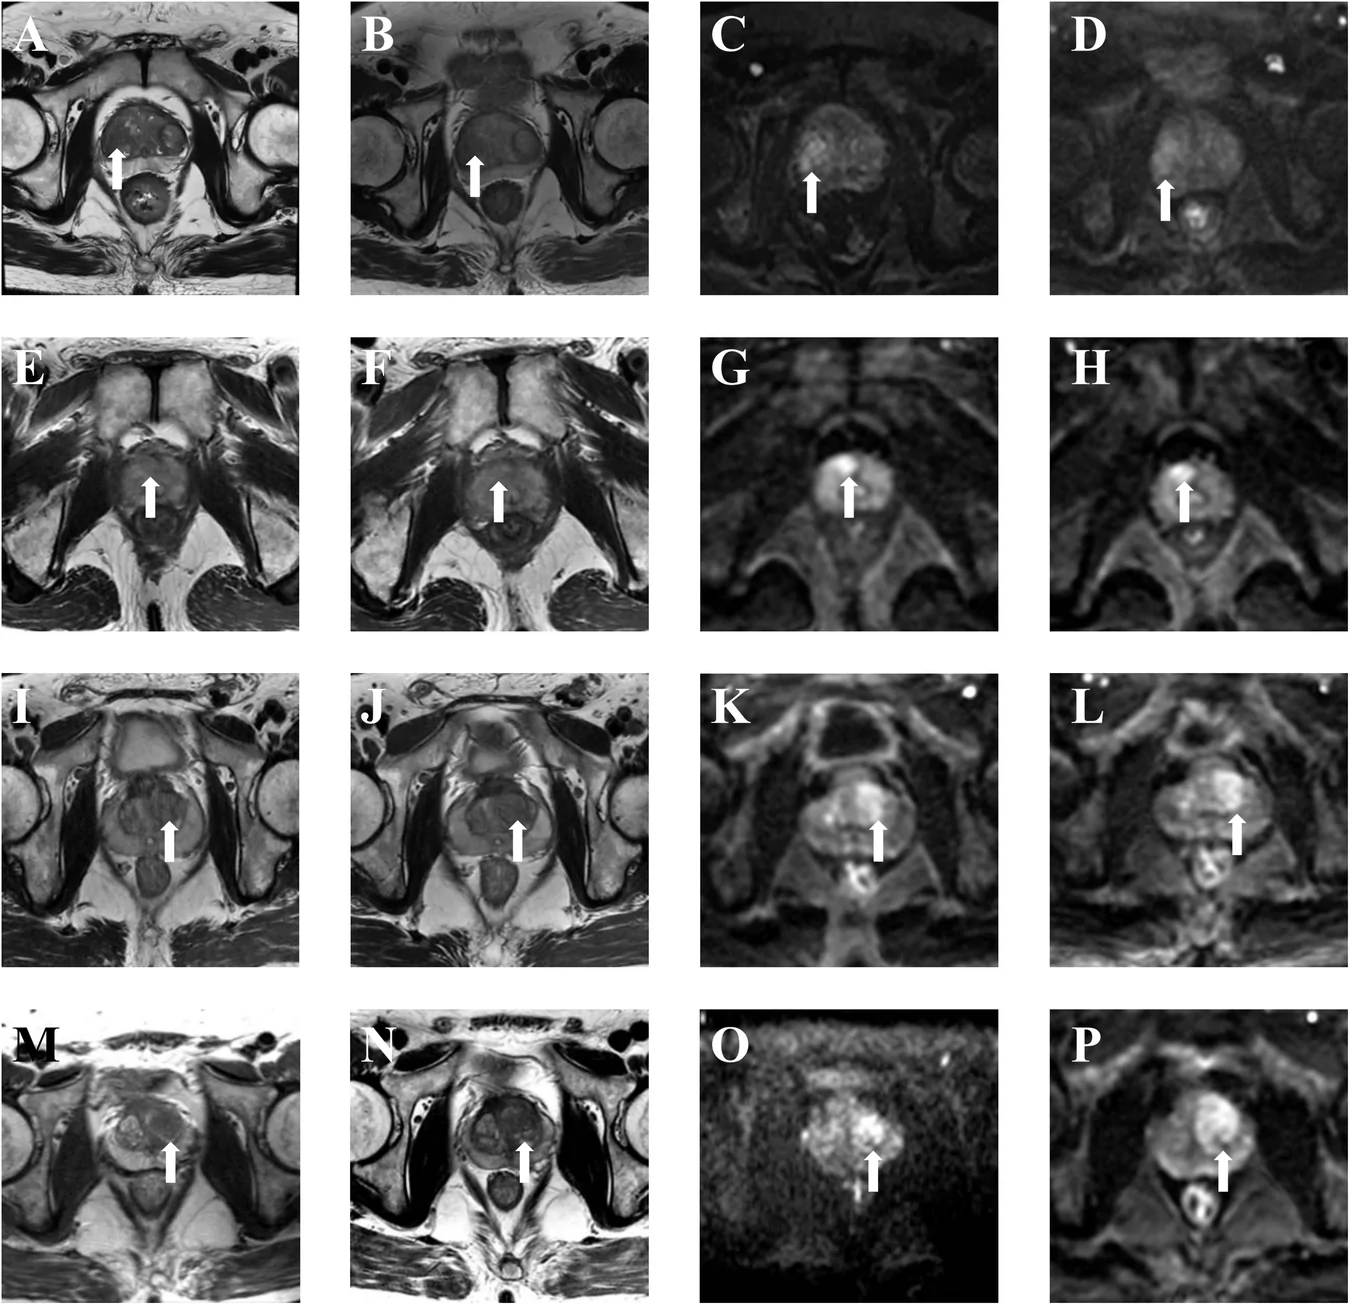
Representative examples of sequential biparametric MRI (bpMRI) assessments and repeat biopsy outcomes categorized by the PRECISE scoring system. All suspected lesions are indicated by arrows. A–D Radiological regression (PRECISE 2). A 65-year-old man with an initial negative prostate biopsy. Baseline MRI (A, C) shows a 2.3-cm PI-RADS 5 lesion in the right transition zone, which is hypointense on axial T2-weighted imaging (T2WI) (A) and hyperintense on diffusion-weighted imaging (DWI) (C). Follow-up MRI at 14 months (B, D) demonstrates a clear reduction in lesion size to 1.6 cm on corresponding T2WI (B) and DWI (D). The lesion was assigned a PRECISE score of 2. Repeat biopsy revealed no evidence of prostate cancer. E–H Radiological stability (PRECISE 3) with clinically significant prostate cancer (csPCa). Sequential MRIs demonstrate a persistently visible 0.8-cm focal lesion in the peripheral zone. The lesion remained unchanged and was scored as PI-RADS 3 on both the baseline (E, G) and follow-up (F, H) scans, yielding a PRECISE score of 3. Repeat targeted biopsy detected csPCa with a Gleason score of 3 + 4. I–L Radiological stability (PRECISE 3) without prostate cancer. Sequential MRIs show a persistent lesion in the transition zone assigned a PI-RADS score of 4 on both baseline (I, K) and follow-up (J, L) examinations. Because of the lack of significant radiological change, it was assigned a PRECISE score of 3. Repeat biopsy was negative for prostate cancer. M–P Radiological progression (PRECISE 4). A 57-year-old man whose initial biopsy indicated only inflammation. Baseline MRI (M, O) reveals a 1.4-cm PI-RADS 3 lesion in the left transition zone, appearing hypointense on T2WI (M) and hyperintense on DWI (O). Follow-up MRI at 22 months (N, P) demonstrates increased focal conspicuity and signal intensity on corresponding T2WI (N) and DWI (P). Both radiologists assigned a PRECISE score of 4. Subsequent targeted biopsy confirmed csPCa with a Gleason score of 4 + 3
